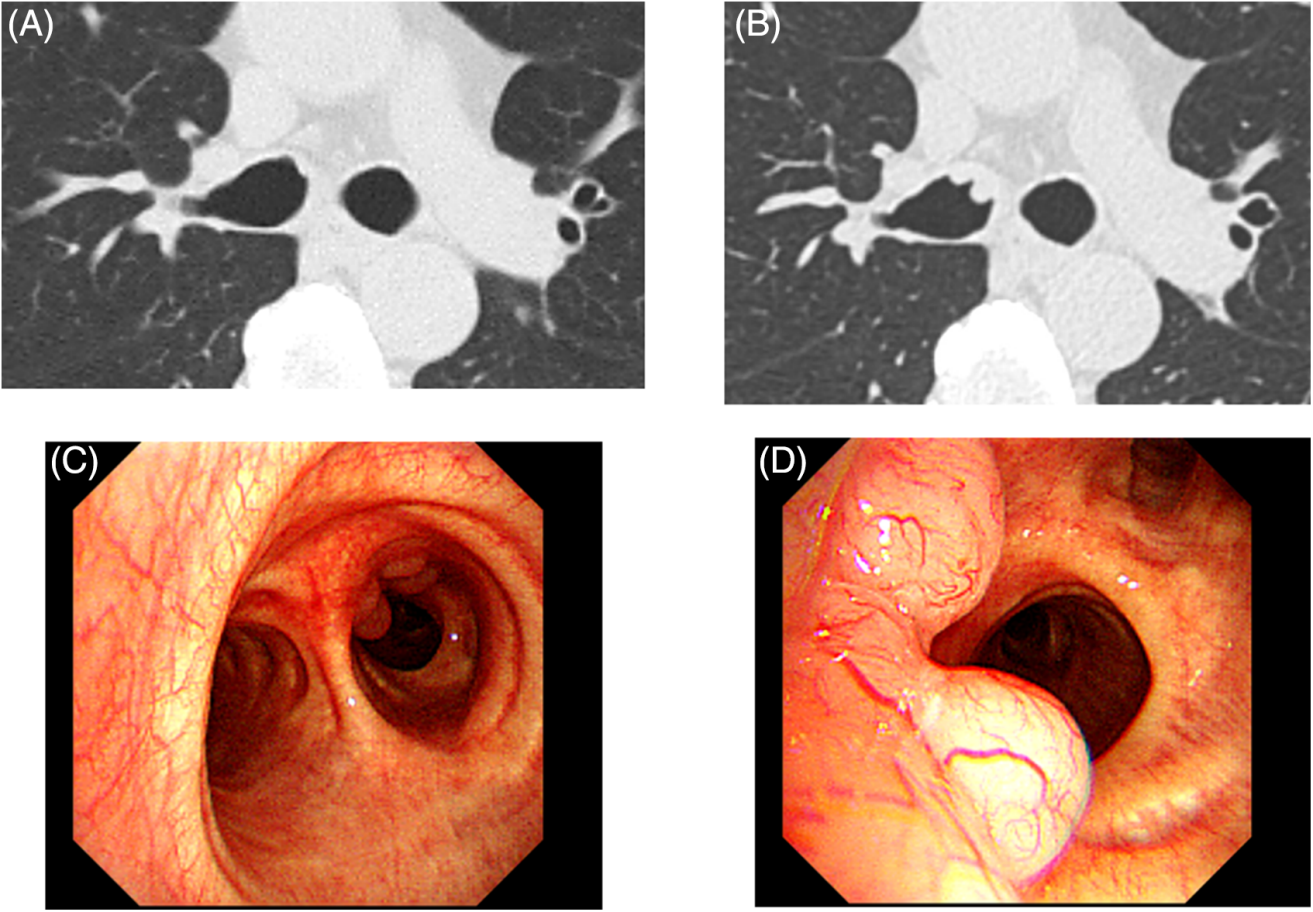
Chest CT (A, B) and Bronchoscopic findings (C, D). Chest CT showed multiple elevated lesions on the ventral side of the right main bronchus just below the tracheal bifurcation(B). Compared to those obtained 3 years previously (A), the lesions increased in size. Bronchoscopy revealed 5 nodular smooth surfaced protrusions on the ventral side of the right mainstem bronchus just below the tracheal bifurcation (C, D). The maximum size was 5 mm in diameter.
